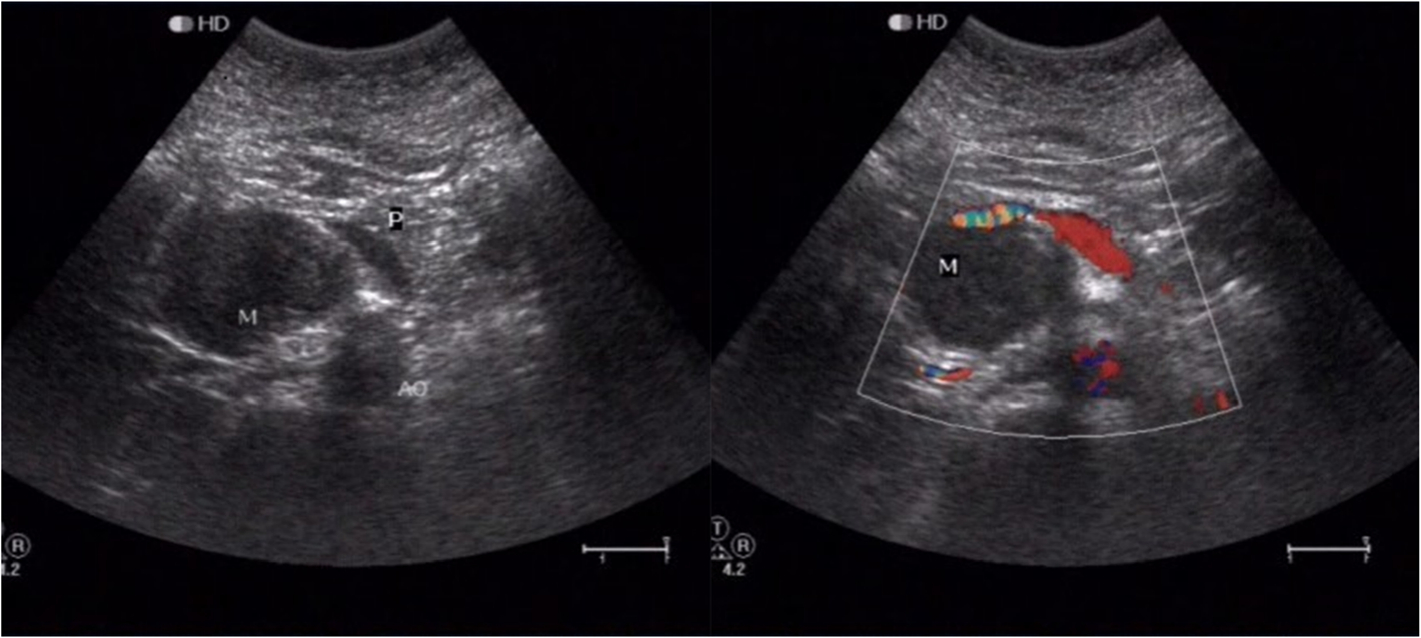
Ultrasound examination demonstrated a hypoechoic mass with irregular shaped, ill-defined and no blood signals in the retroperitoneal space with uncertain origin (P, pancreas; AO: abdominal aorta)
